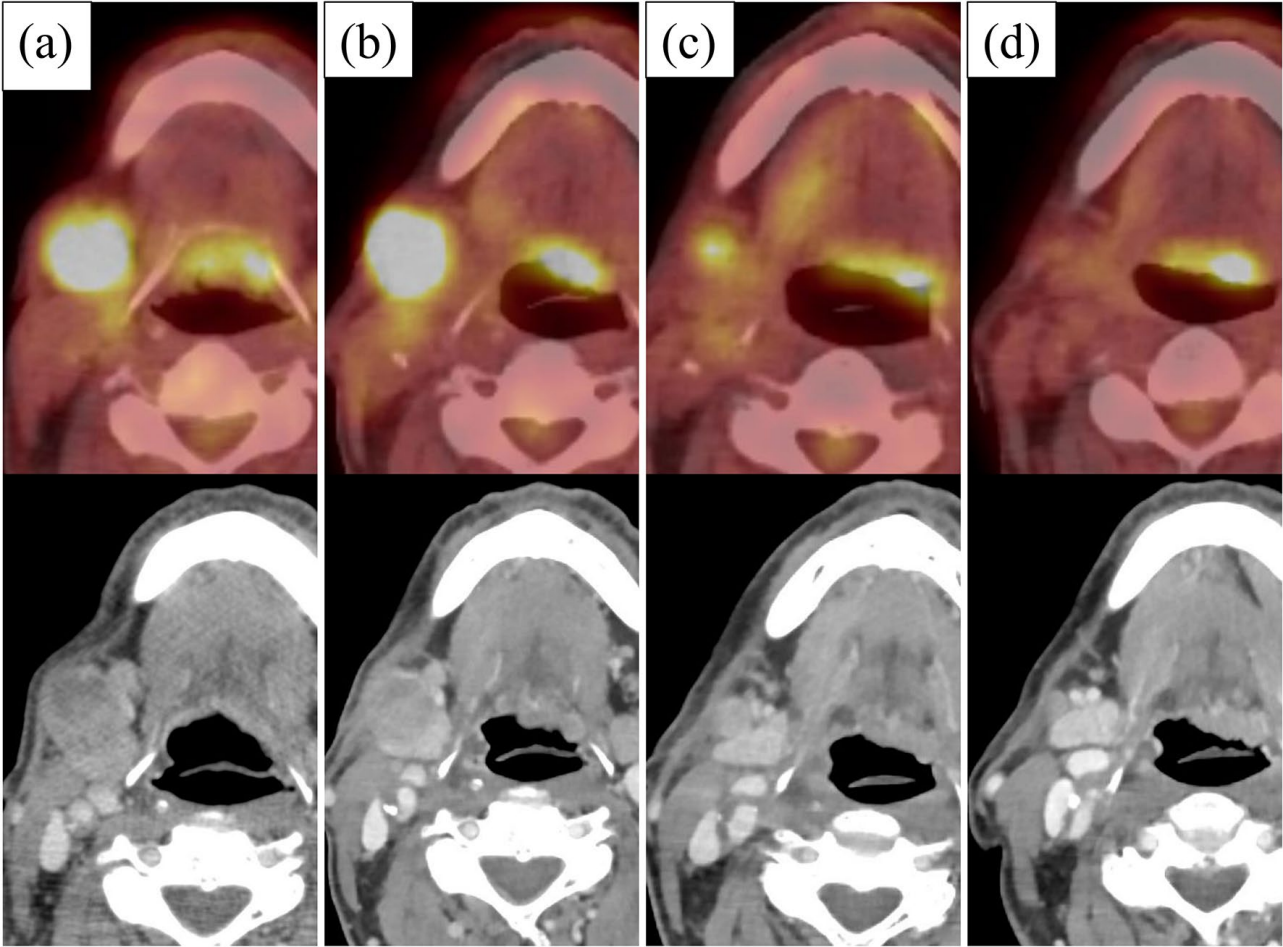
Contrast-enhanced computed tomography and positron emission tomography findings after definitive proton beam therapy. a At pretreatment, the tumor had a diameter of 2.7 cm and fluorine-18-2-deoxy-D-glucose (FDG) accumulation. b At 3 months after proton beam therapy initiation, the tumor diameter was reduced from 2.7 to 2.5 cm, but the FDG accumulation was not reduced. c At 6 months, the tumor diameter had shrunk further to 1.3 cm, and the FDG accumulation had diminished. The lymph nodes in the right neck had also shrunk. d At 12 months, the tumor diameter was further reduced to 0.7 cm, and the FDG accumulation was no longer apparent
